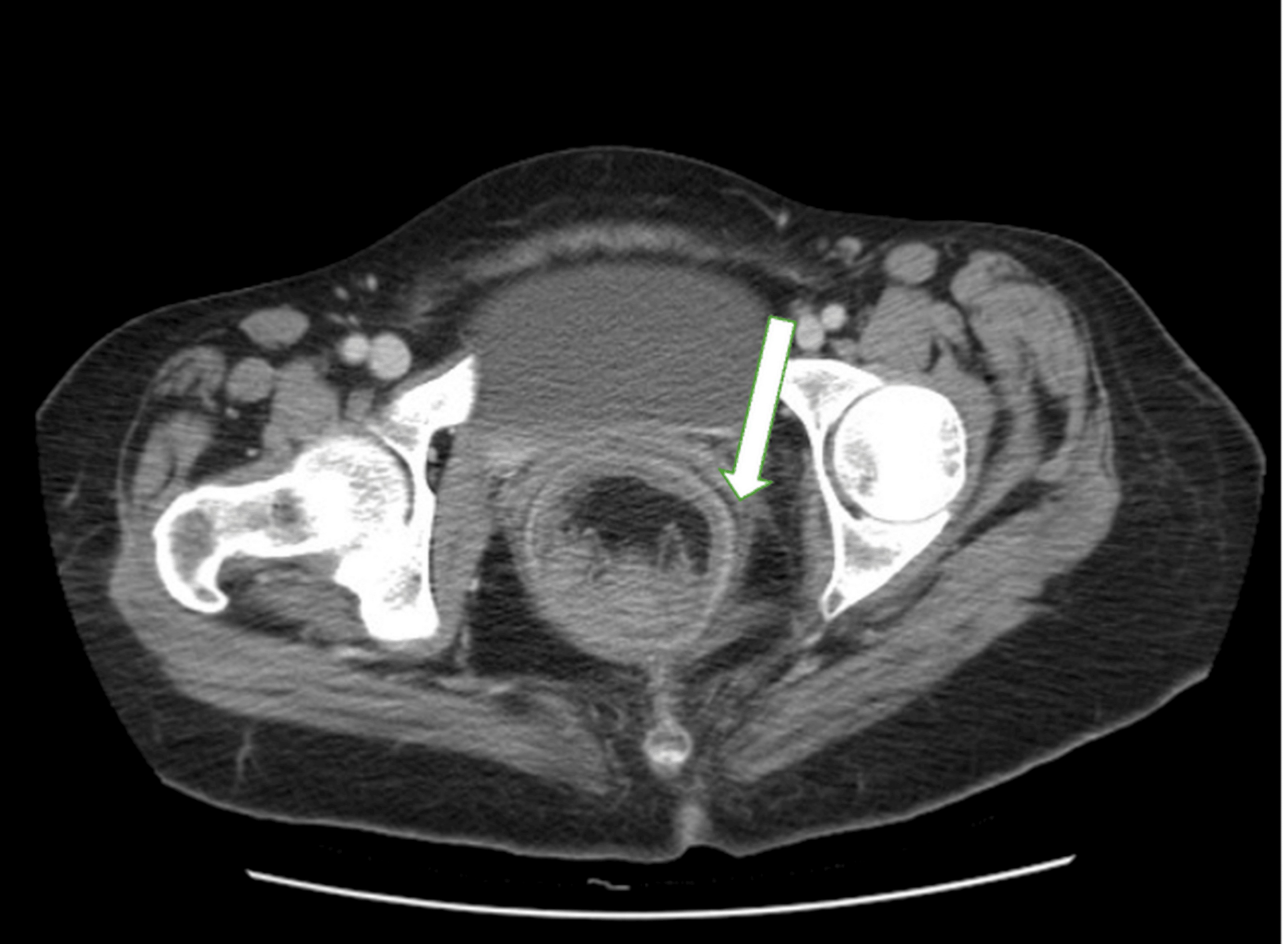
CT abdomen and pelvis, axial view, showing the classic “target sign” of rectosigmoid intussusception (arrow)
